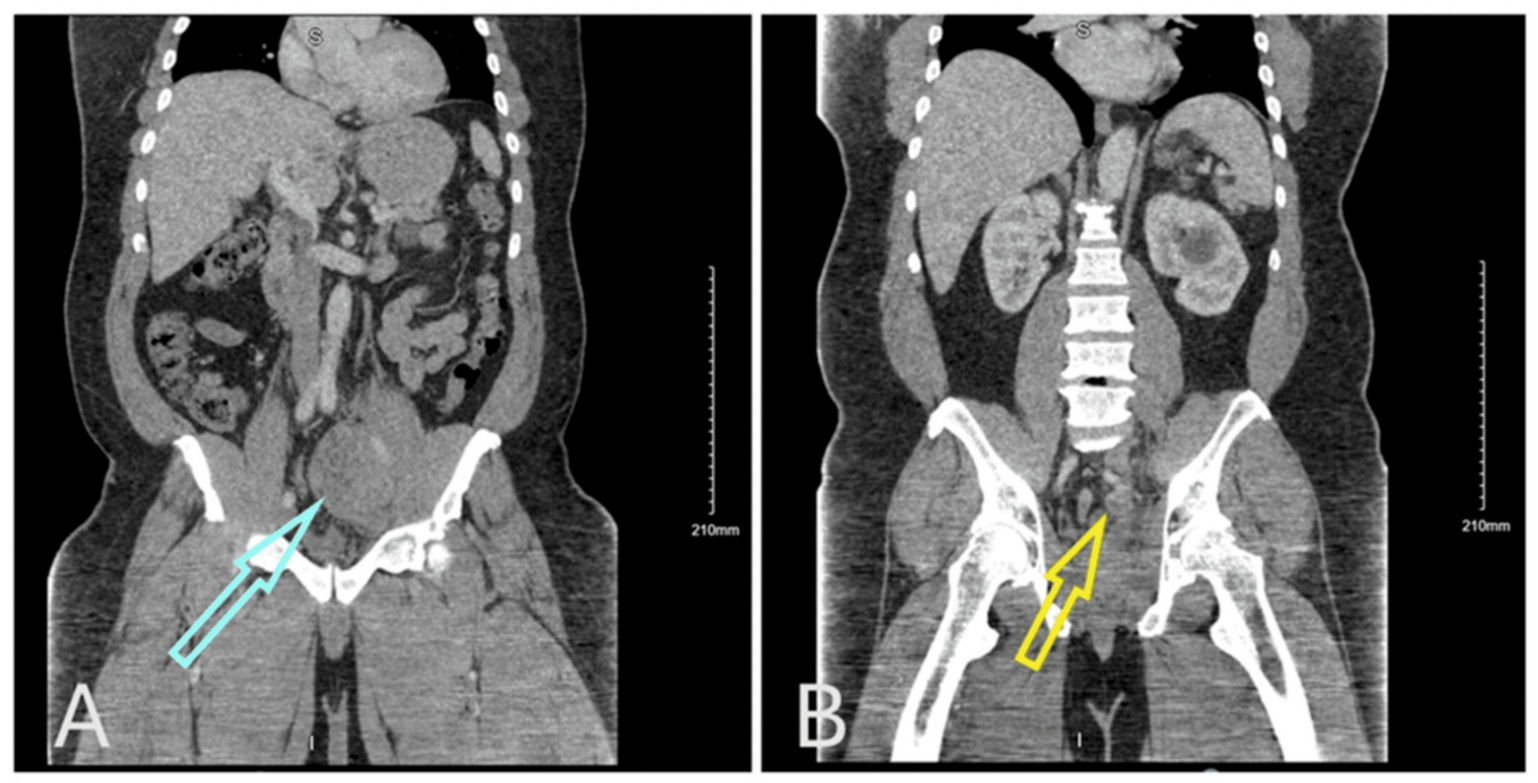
CT scan of the abdomen and pelvis. (A) A heterogeneous mass measuring 9.5 cm × 8.4 cm × 7.5 cm in the left lower quadrant (blue arrow), encasing the left ureter. (B) A smaller heterogeneous mass measuring 2.4 cm × 2.6 cm × 2.2 cm (yellow arrow) inferior to the larger mass. CT: computed tomography
